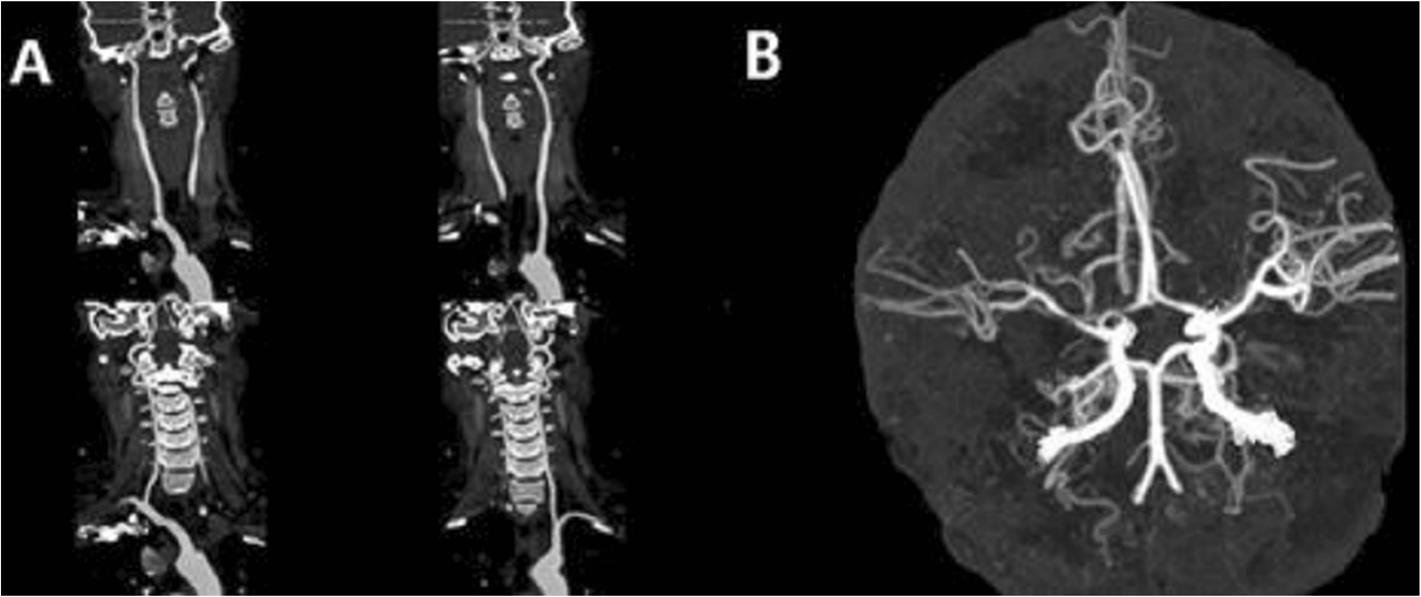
Brain CTA after six days of onset. a No abnormal carotid artery was found. b Stenosis was found in the M1 segment of the right middle cerebral artery and the distal branches were significantly less than those of the opposite side
